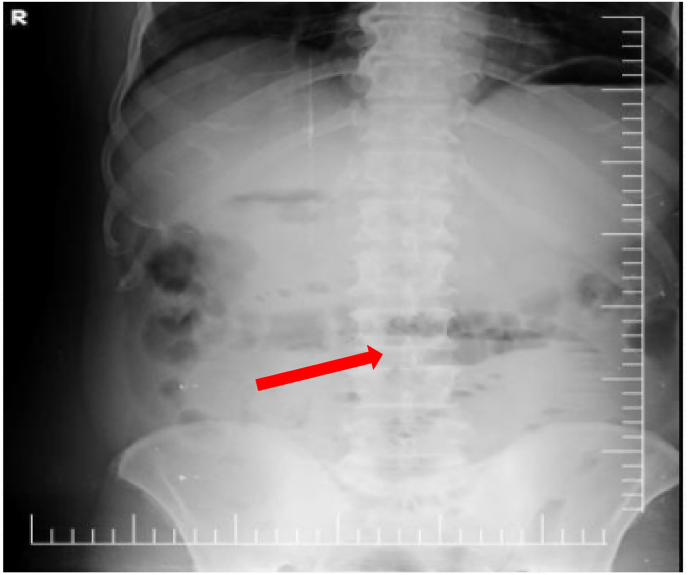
Erect abdominal plain film shows mild dilated small bowel loops with air-fluid levels in bowel and stomach.
